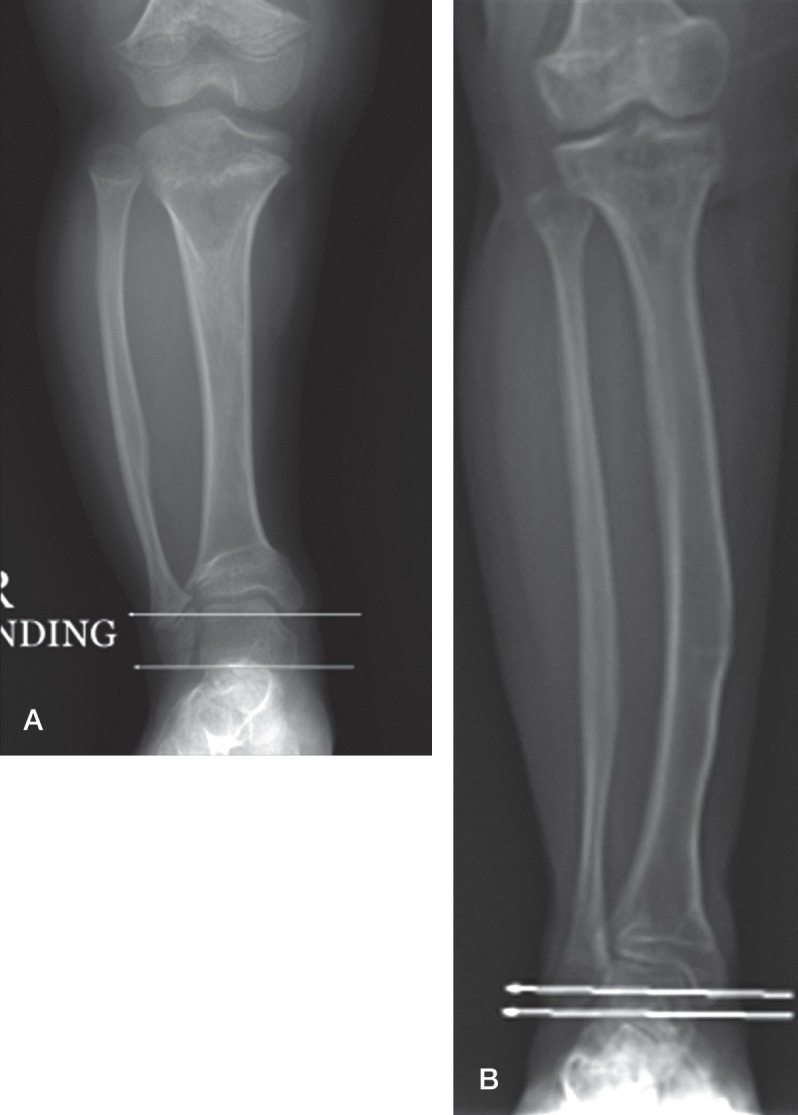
A. An 11-year-old girl with achondroplasia. Preoperative standing. The proximal line shows the level of the tip of the medial malleolus while the distal line denotes the level of the tip of the lateral malleolus. B. Proximal migration of the distal fibula with valgus deformity of the right ankle. Proximal migration of the distal fibula was measured by comparing the amount of the lateral malleolus projecting distal to the medial malleolus on preoperative and postoperative radiographs.
